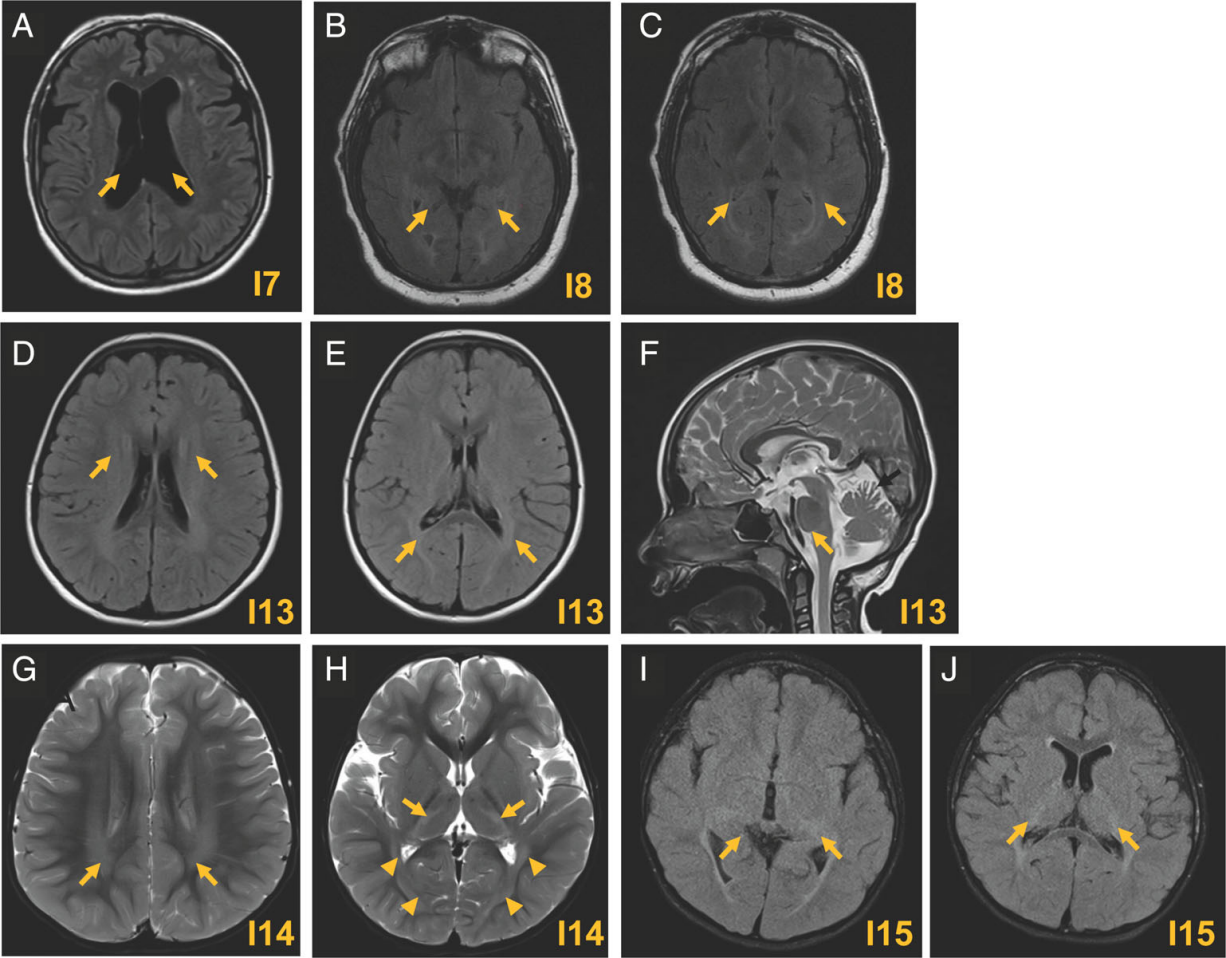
Cerebral MRI from individuals carrying the p.(Ala363Val), the p.(Asn369Ser), and the p.(Glu478del) variants. (A) Axial FLAIR MRI image from individual I7 show atrophy and an increase of ventricular spaces (arrows). Individual I7 carries the p.(Ala363Val) variant. (B, C) Axial brain MRI images show mild T2 signal changes in the periventricular white matter posteriorly in individual I8, carrying the p.(Asn369Ser) variant. (D–H) Axial MRI scans of individuals carrying the p.(Glu478del). (D–F) Axial FLAIR MRI images of individual I13 show periventricular white matter abnormalities. (F) Sagittal T2‐weighted image shows superior vermis atrophy (arrow C). (G, H) Axial T2‐weighted image through the centrum semiovale of individual I14 demonstrates a hyperintense signal involving the periventricular white matter with posterior predominance (arrows D). T2‐weighted signal hyperintensity is also present in the ventrolateral thalami (E arrows), as well as the optic radiations and subcortical occipital white matter (E arrow heads). (J, K) Axial T2‐weight images demonstrate posterior mild diffuse periventricular hyperintensity signal abnormality that extended into the posterior capsule into the thalami bilaterally in individual I15. FLAIR = fluid‐attenuated inversion recovery; MRI = magnetic resonance imaging. [Color figure can be viewed at www.annalsofneurology.org]
